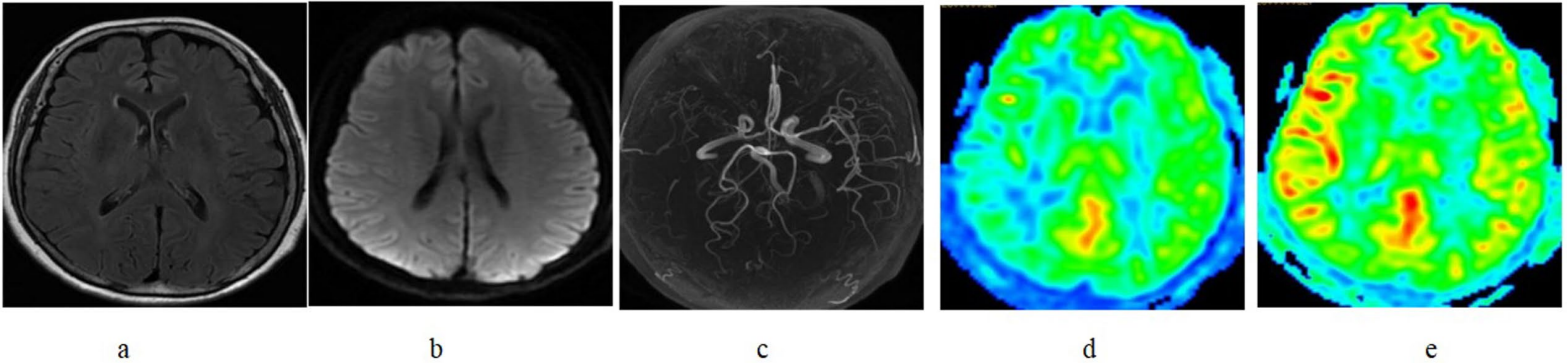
FLAIR vascular hyperintensity absent in a patient with right middle cerebral artery occlusion. (a) FLAIR vascular hyperintensity absent in T2 FLAIR and (b) DWI demonstrate negative finding. (c) MRA showed occlusion of the right MCA M1. The area of the right MCA demonstrated to be hypoperfused on ASLwith a PLD of 1525 ms (d) but improved on ASL with a PLD of 2525 ms (e).
